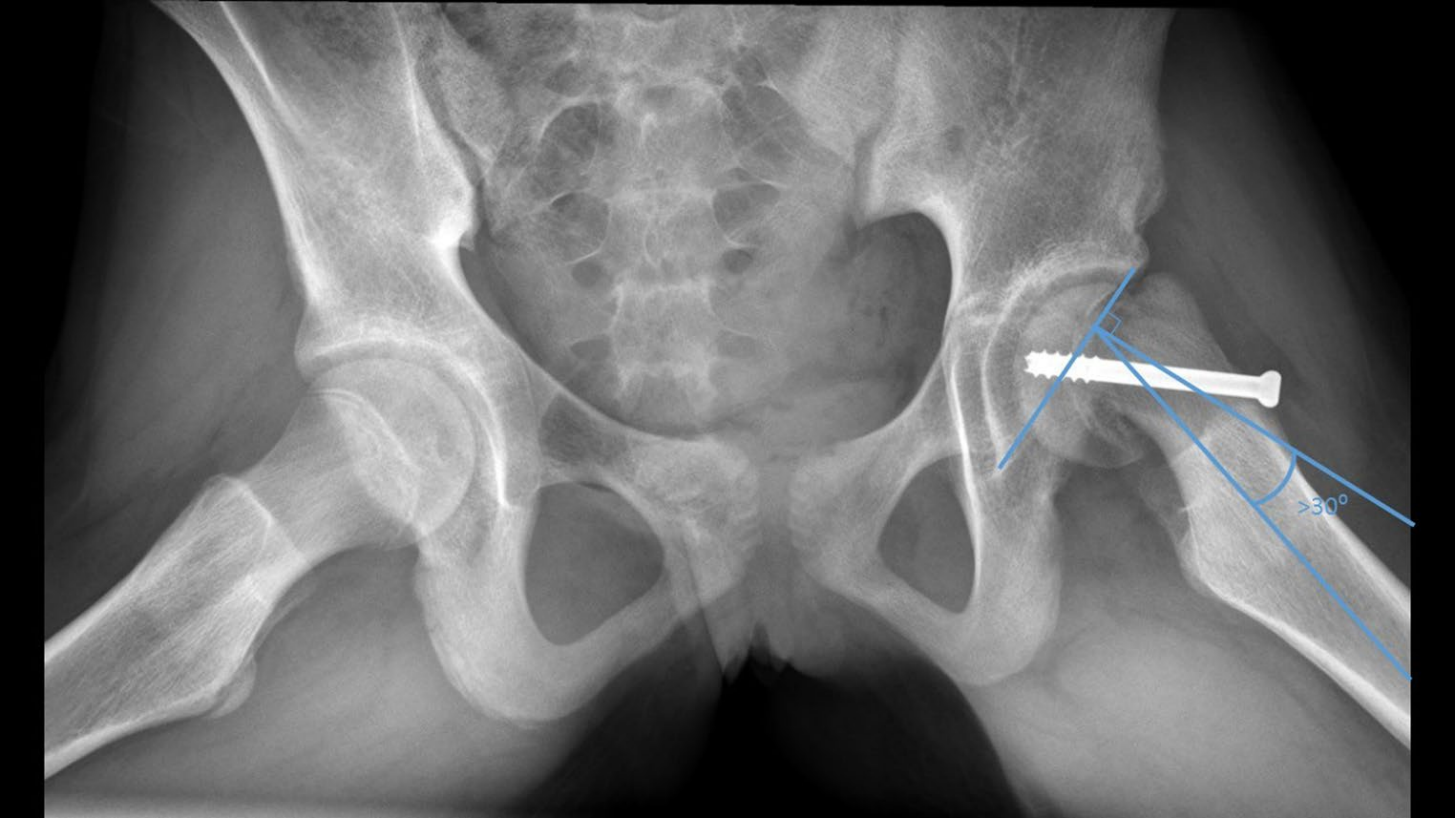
Example of an X-ray with the measurement of the Southwick angle
